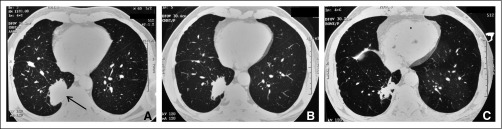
High-resolution computed tomography of the thorax at (A) baseline showing the right lower lobe (RLL) mass reaching up to the pleura. Multiple random nodules, some of which were subpleural, were also present in right lung, as was right-sided pleural effusion. (B) After 4 cycles of chemotherapy, showing reduction in the size of the RLL mass, as well as the number and size of the lung nodules. (C) During erlotinib and bevacizumab treatment, showing partial response in the form of decrease in size of the RLL mass, as well as size and number of lung nodules.
